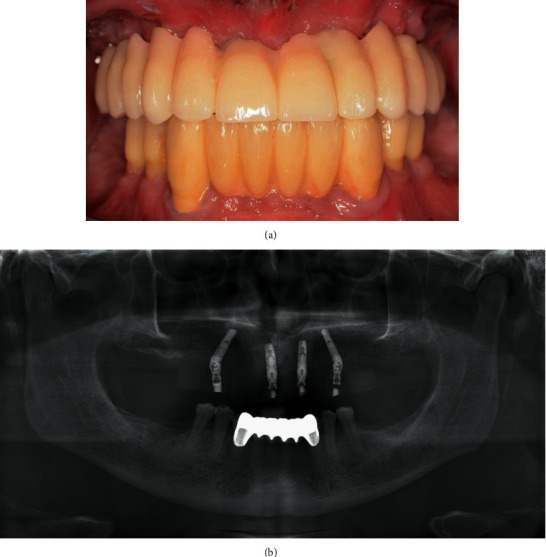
(a) Frontal view of the final inserted provisional FPD. (b) Orthopantomogram to control the implant position.
